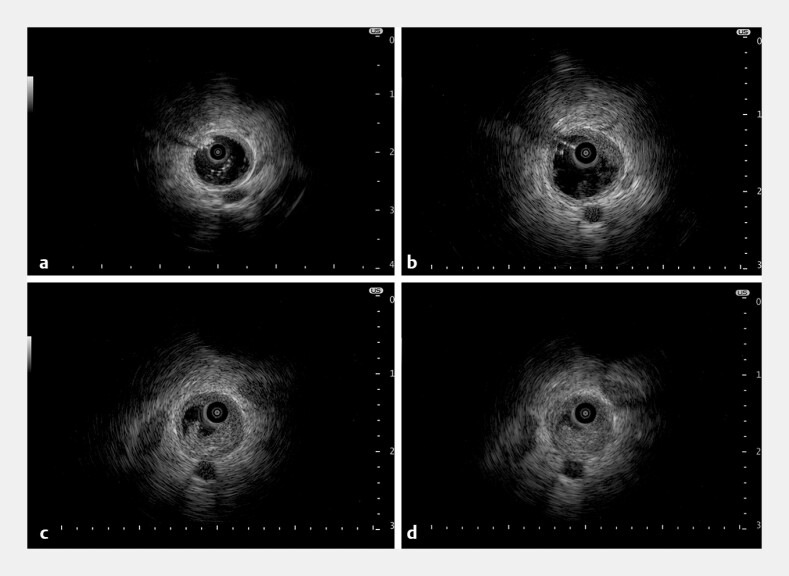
Detailed intraductal ultrasonography images showing:
